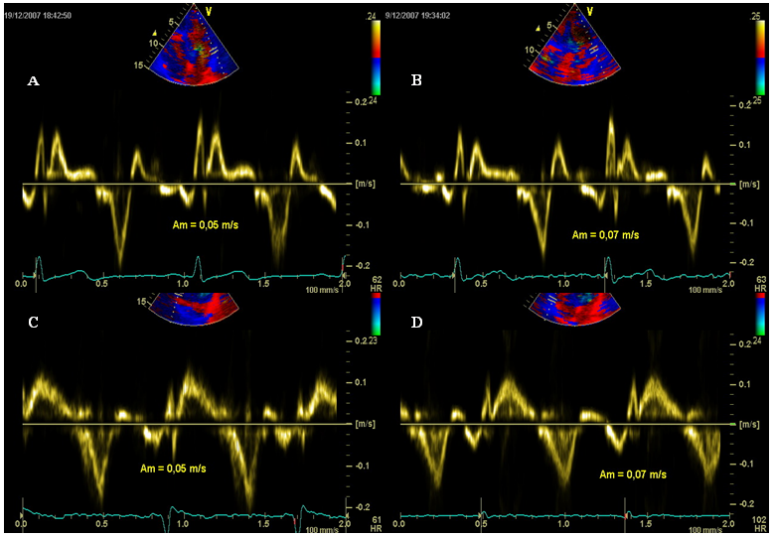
Tissue Doppler pattern of mitral and tricuspid annulus motion at baseline (panels A-C) and after coffeine assumption followed by cigarette smoking (panels B-D), showing an increase in both mitral and tricuspid peak A_m _velocities.
